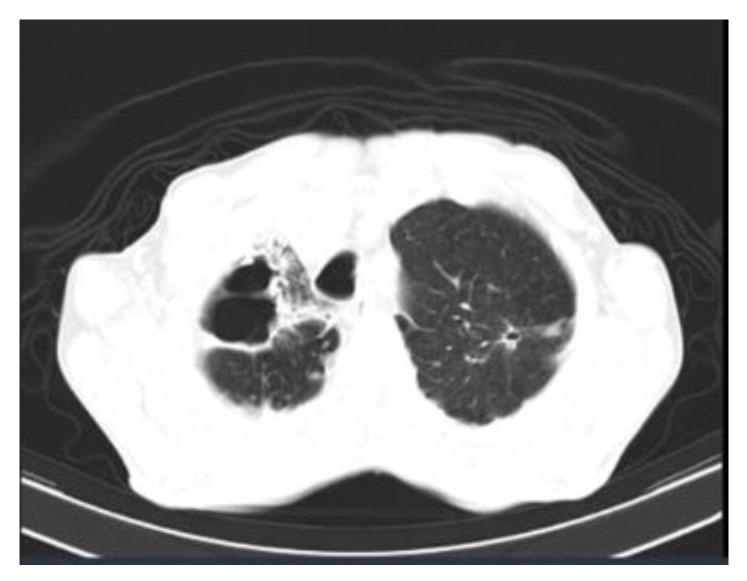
Pulmonary sporotrichosis in a 63-year-old man. Nonenhanced chest computed tomography showing, among other alterations, thick-walled cavities with irregular margins in the right lower lobe associated with architectural distortion, fibrotic opacities, and bronchiectasis. There are also linear opacities with a fibrotic appearance and bronchiectasis in the apicoposterior segment of the left upper lobe.
